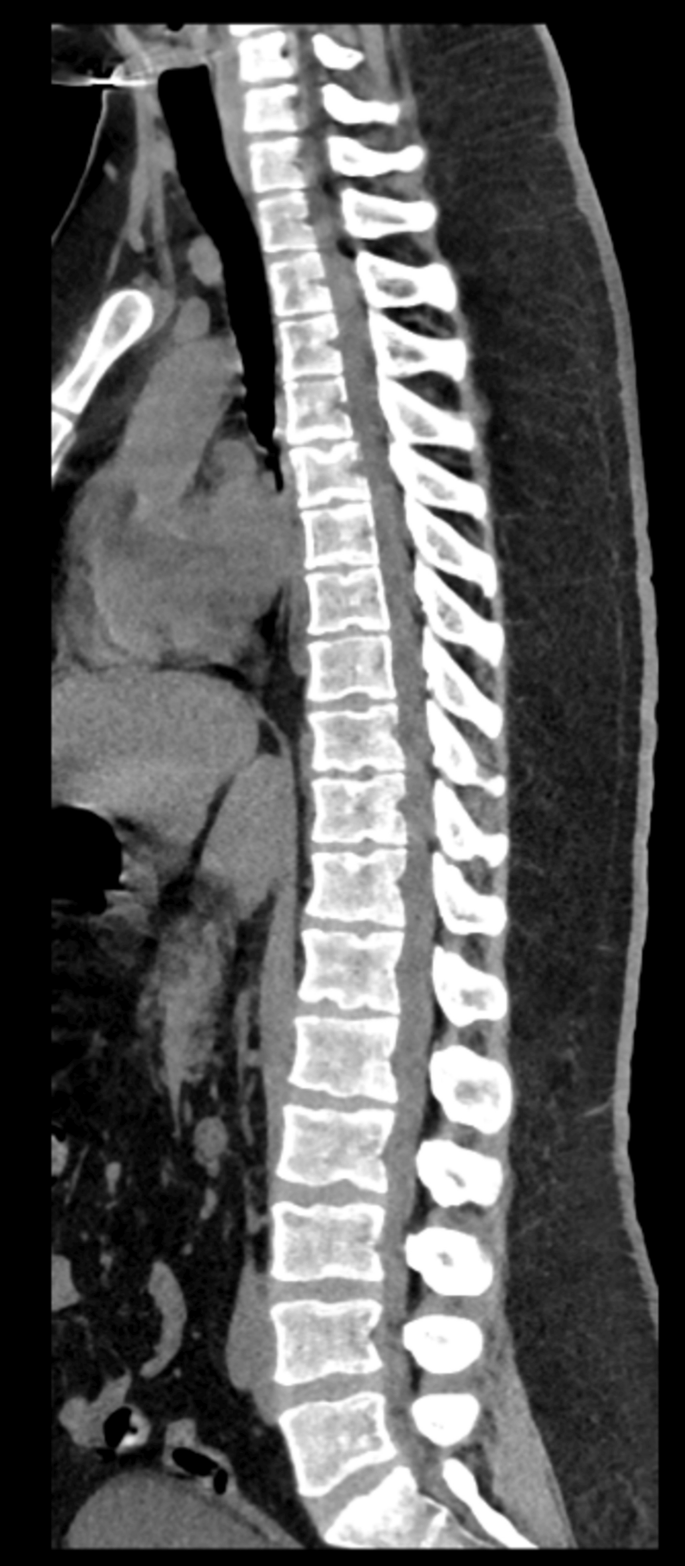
CT scans revealing no abnormal findings from the imaging of the spine.
